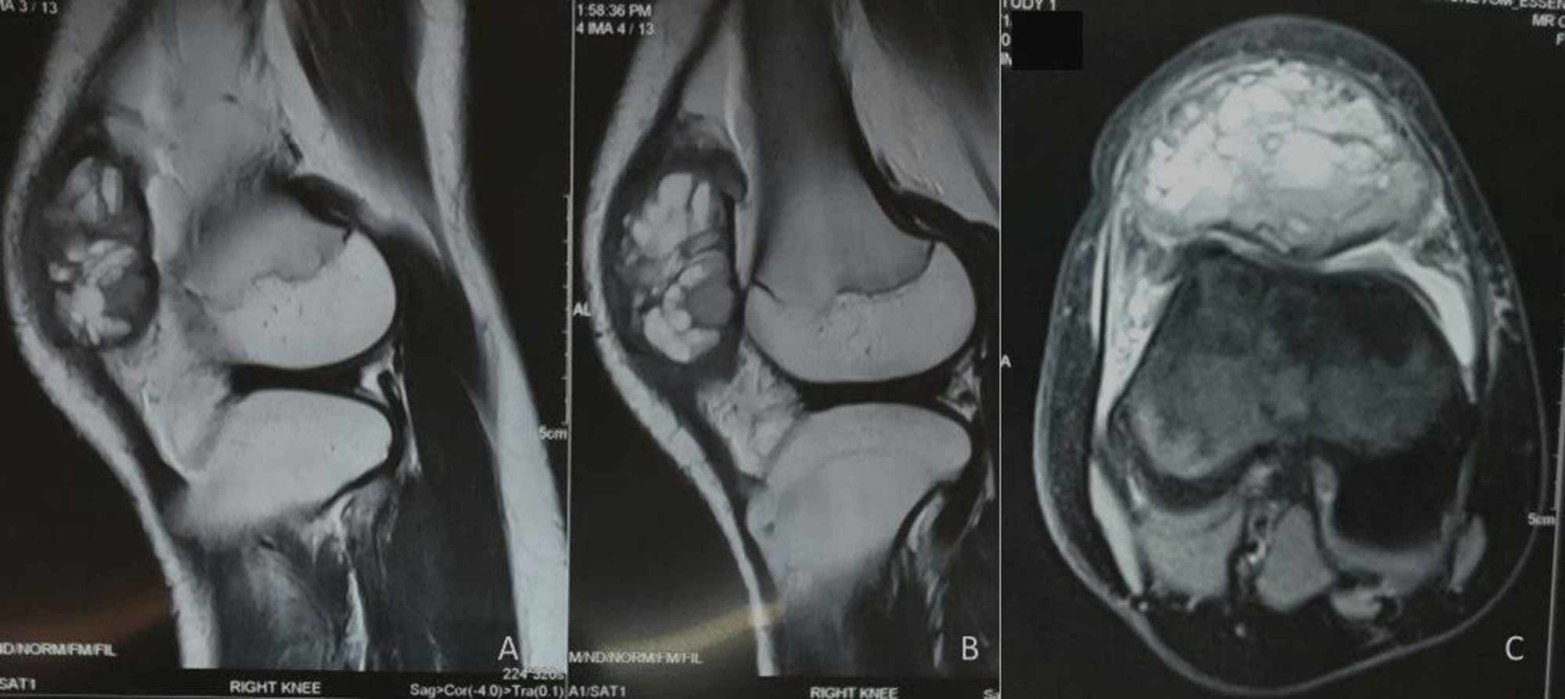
Sagittal (A, B) and axial cut sections show T2-weighted hyperintense signal with no involvement of overlying soft tissue
